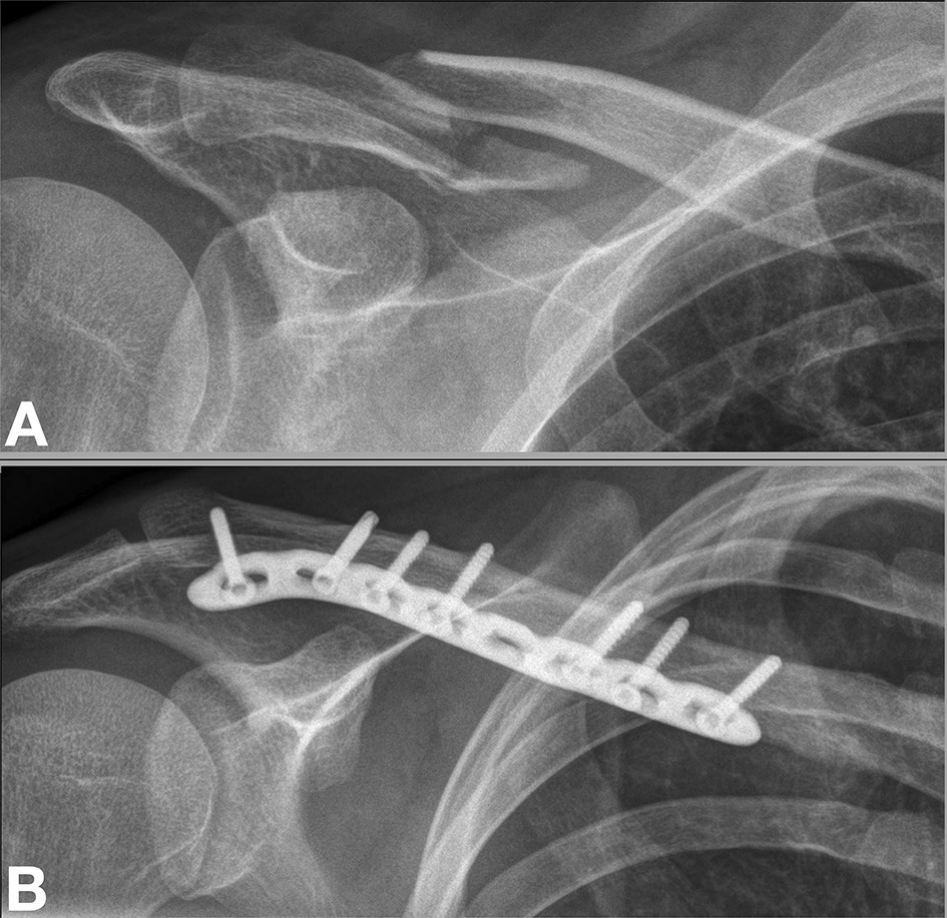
Radiographic anteroposterior images (right shoulder) of an 18-year-old male following a fall while playing soccer demonstrating a midshaft clavicle fracture and fracture fixation with an anteroinferior plate. (A) Displaced midshaft clavicle fracture, (B) plate fixation with an anteroinferior plate.
